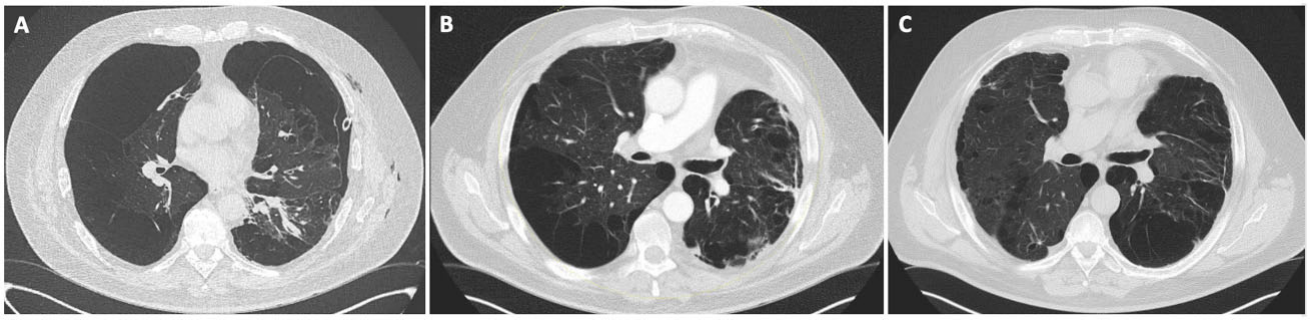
Chest CT following talc pleurodesis (A). Chest CT 1 month after left lung volume reduction surgery (B). Chest CT 1 month after right lung volume reduction surgery (C).
